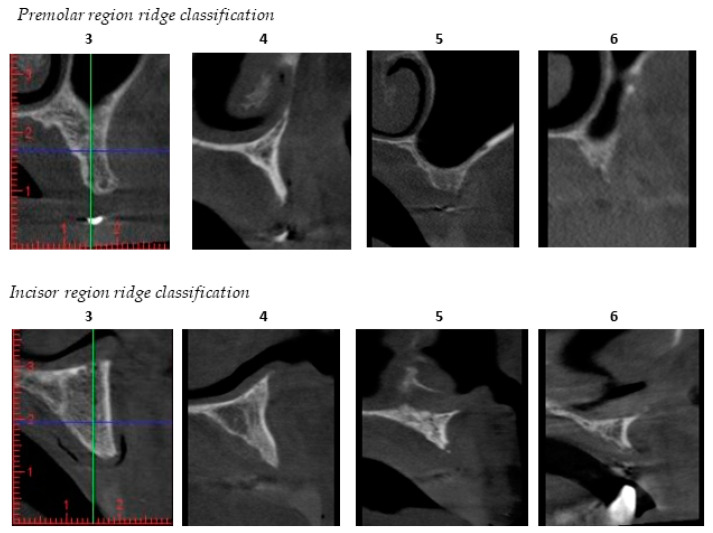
Cawood–Howell classification [23] of the maxillary alveolar ridge applied to cone-beam computerized tomography (CBCT) images in the patient population studied. This classification describes a progressive bone resorption pattern in 7 scores; score 1 being where a tooth is still present; and score 7 being extensive alveolar bone resorption. Score 3 and 4 represents bone which is sufficient in height, but too narrow in the crestal and midfacial zone (knife edge). Scores 5 to 6 show insufficient bone height and/or width to allow conventional implant placement without additional grafting procedures. Two examiners, the treating surgeon (L.V.D.) and the oral radiologist (G.H.), assessed all CBCTs independently to describe the quantity of available bone.
